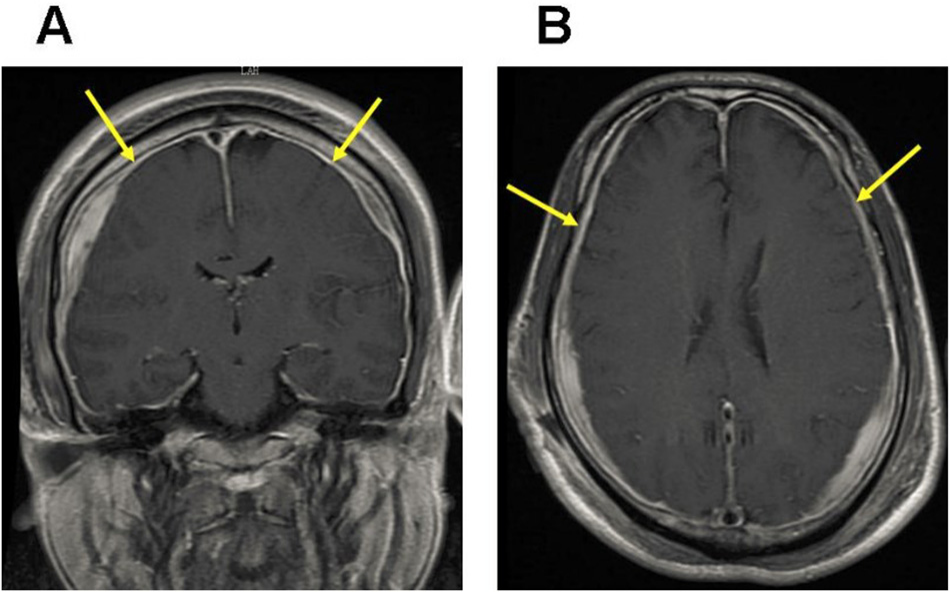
Typical MRI manifestation of dural enhancement. T1-weighted MRIs with gadolinium enhancement shows dural enhancement in coronal (A) and horizontal (B) images (arrows).
